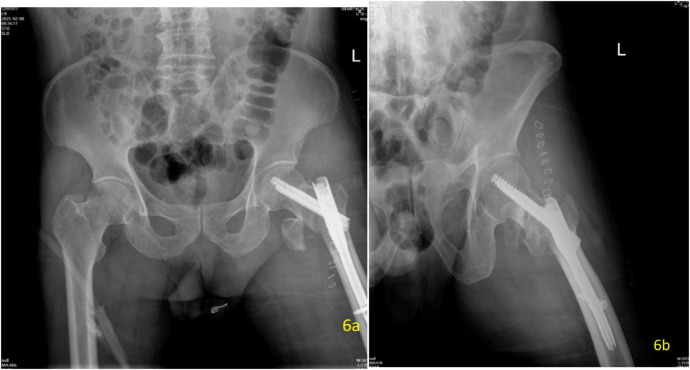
Two-panel postoperative x-ray series of the left hip on day 1. The left image (a, anteroposterior view) and the right image (b, lateral view) confirm satisfactory fracture reduction and positioning of the internal fixation implant.
